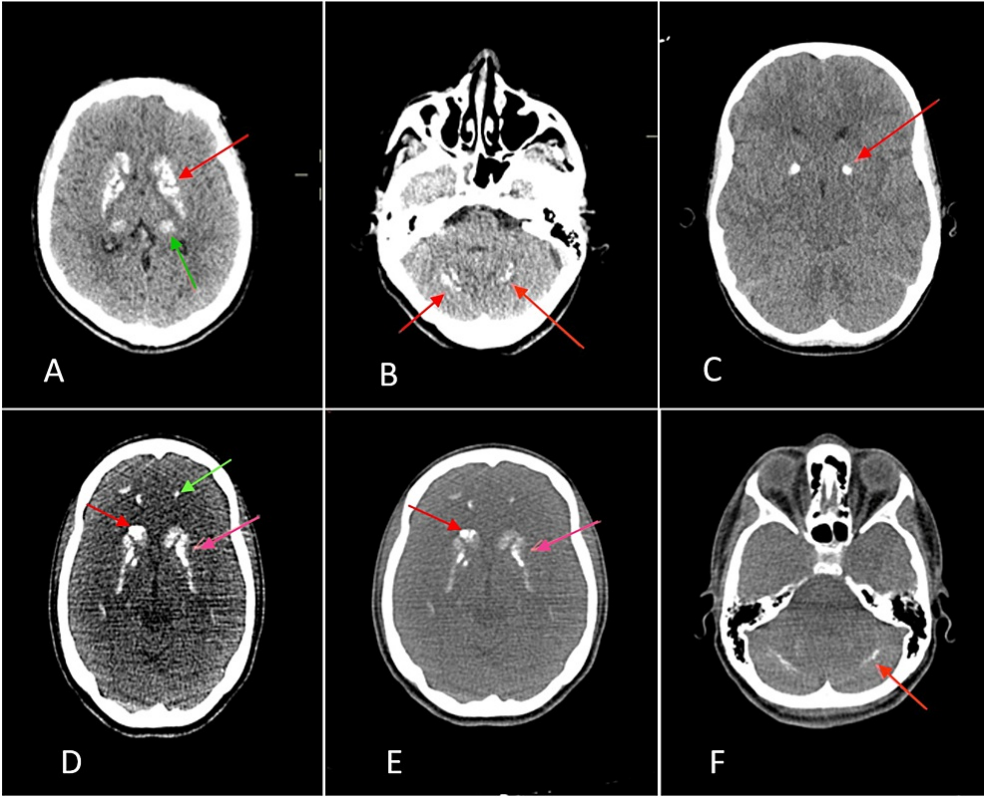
Brain CT scan showing bilateral calcifications of the basal ganglia A. Calcifications of the thalami (green arrow) lenticular and caudate nuclei (red arrow) B. Calcifications of the dentate nuclei (red arrow) C. Calcifications of the lenticular nuclei (red arrow) D. Calcifications of the caudate nuclei (red arrow), subcortical structures (green arrow), and lenticular nuclei (pink arrow) E. Calcifications of the lenticular (pink arrow) and caudate nuclei (red arrow) F. Calcifications of the dentate nuclei (red arrow)
